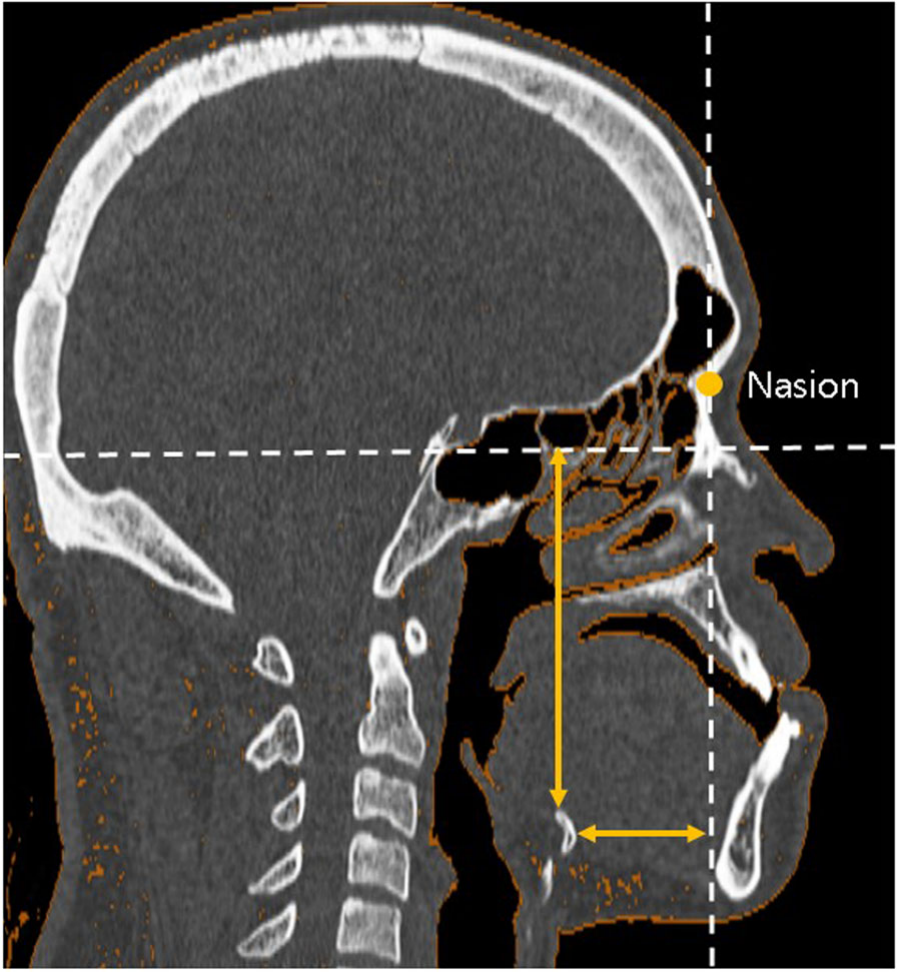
Vertical and horizontal positioning of the hyoid bone was measured in CT (mm). The *x*-axis was defined as the line tangent to the inferior point of the sella turcica and parallel to the FH plane, while the *y*-axis was defined as the line passing through the nasion and perpendicular to the *x*-axis
